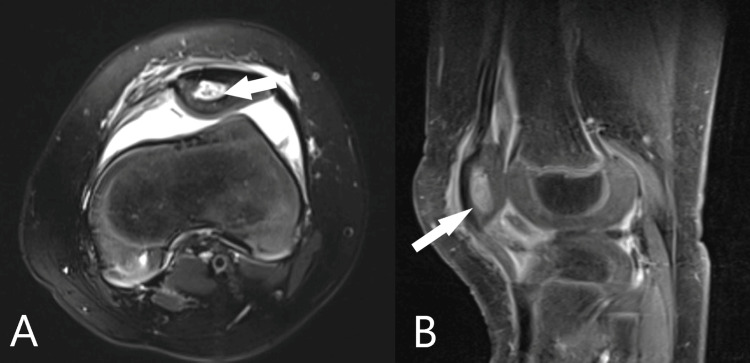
Magnetic resonance imaging with axial (A) and sagittal (B) cuts of the left knee showing patellar osteomyelitis with associated cortical destruction (arrow in B) and a small communicating sinus tract (arrow in A)
